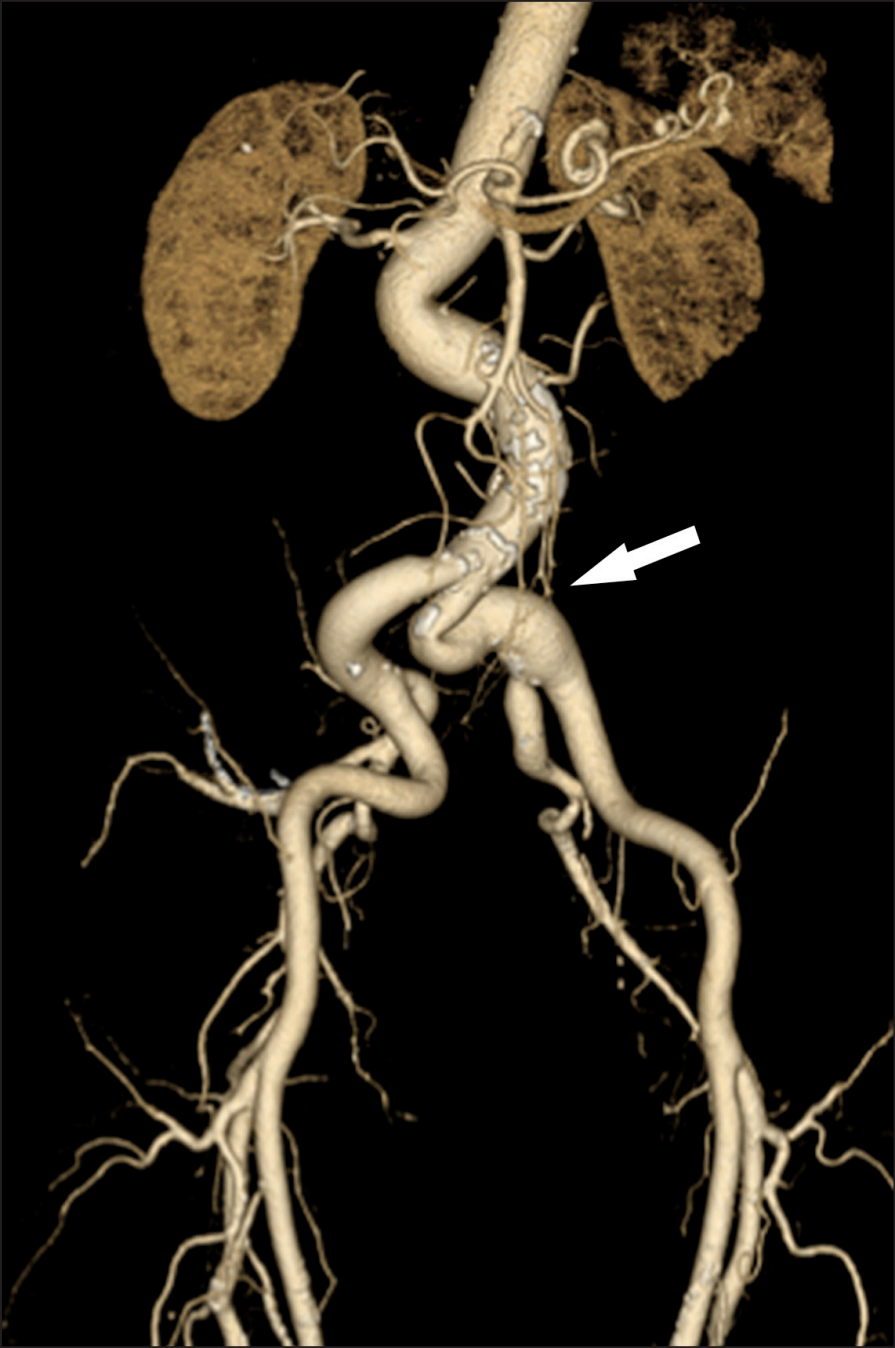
Inadequate access route patency. Volume-rendered CT image obtained in a 74-year-old patient shows general tortuosity of the aortoiliac arterial vessels, a finding that is most prominent in the left common iliac artery (arrow). This pronounced tortuosity will increase procedural risk, in this case making the right iliac arteries a better (although not perfect) option.
